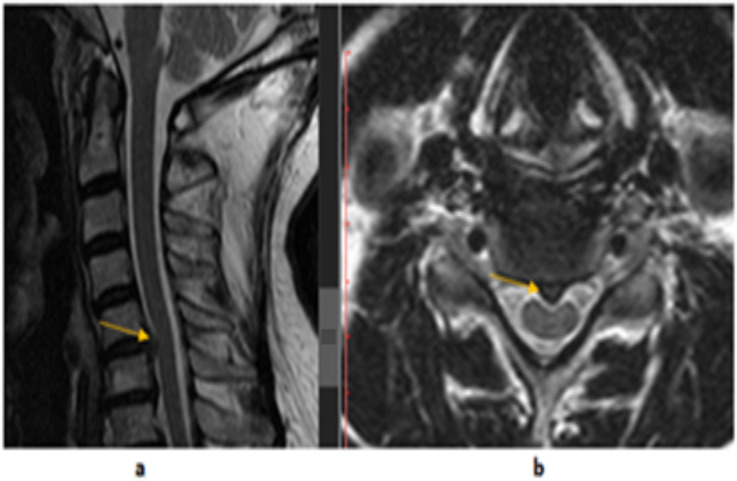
A 52-year-old female patient, (a) T2W sagittal and (b) T2W axial, cervical spine MRI images. A C5-C6 level posterior central disc protrusion (arrows), which cause spinal canal stenosis and cord compression. A decreased signal of the intervertebral discs and straightening of cervical spine alignment are demonstrated
